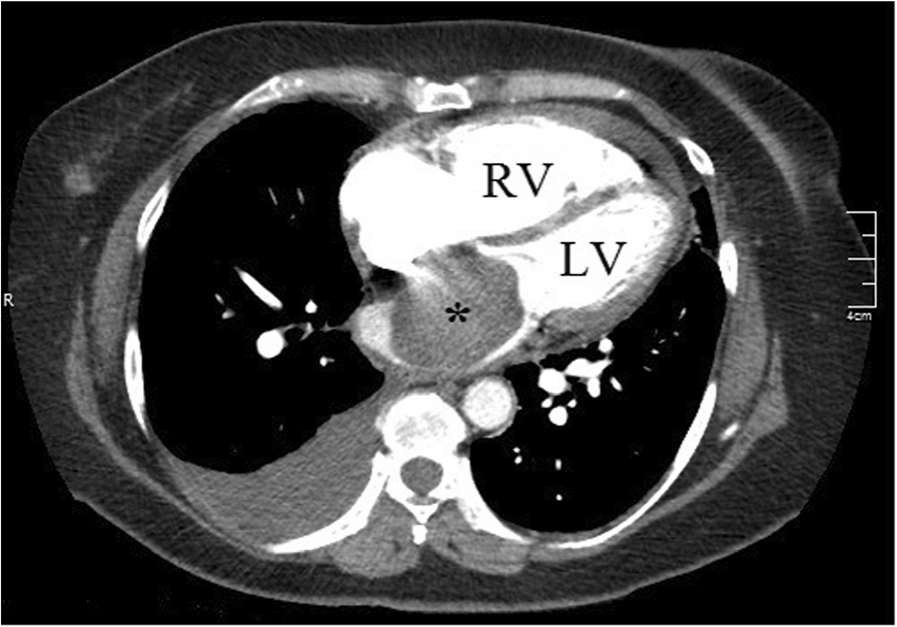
Computed tomography angiogram of the chest exhibiting a left atrial filling defect (asterisk). Note the size of the RV in relation to the LV.
